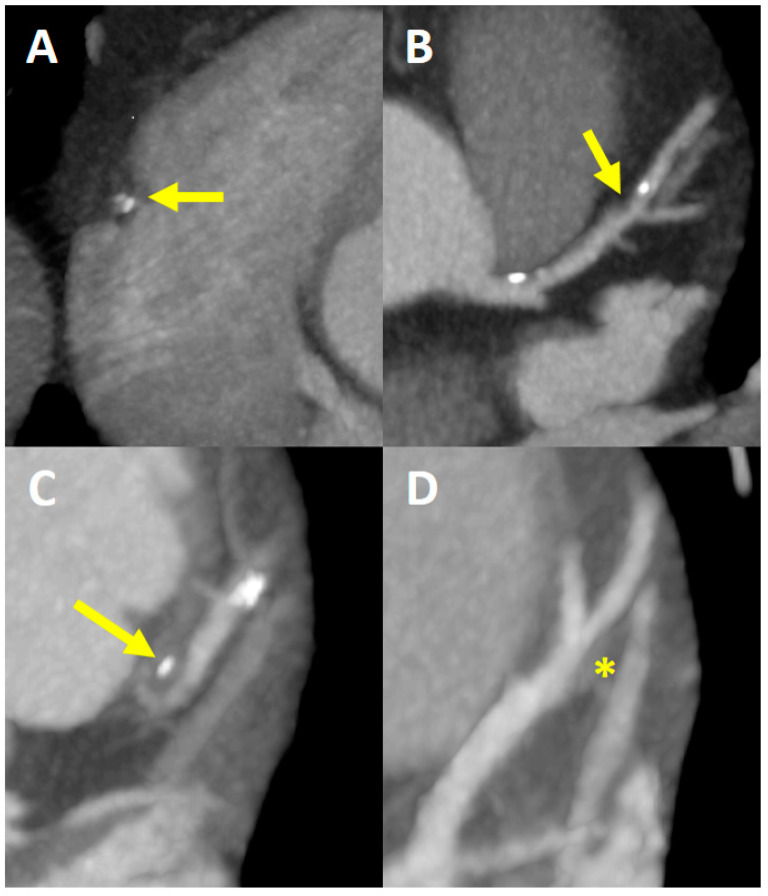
Representative images of high-risk plaque features on coronary computed tomography angiography (CCTA), including the napkin-ring sign ((A) with arrow), low-attenuation plaque (≤30 HU) ((B) with arrow), spotty calcification (<3 mm) ((C) with arrow), and positive remodeling (remodeling index ≥ 1.1) ((D) with asterisk). These imaging characteristics are used to define high-risk coronary plaques (HRP) in this study and serve as the primary outcome linked to NPS.
